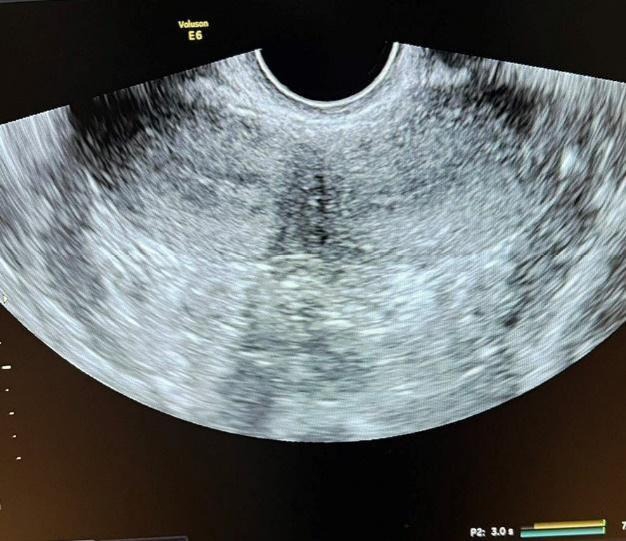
Uterine didelphys on ultrasound.
